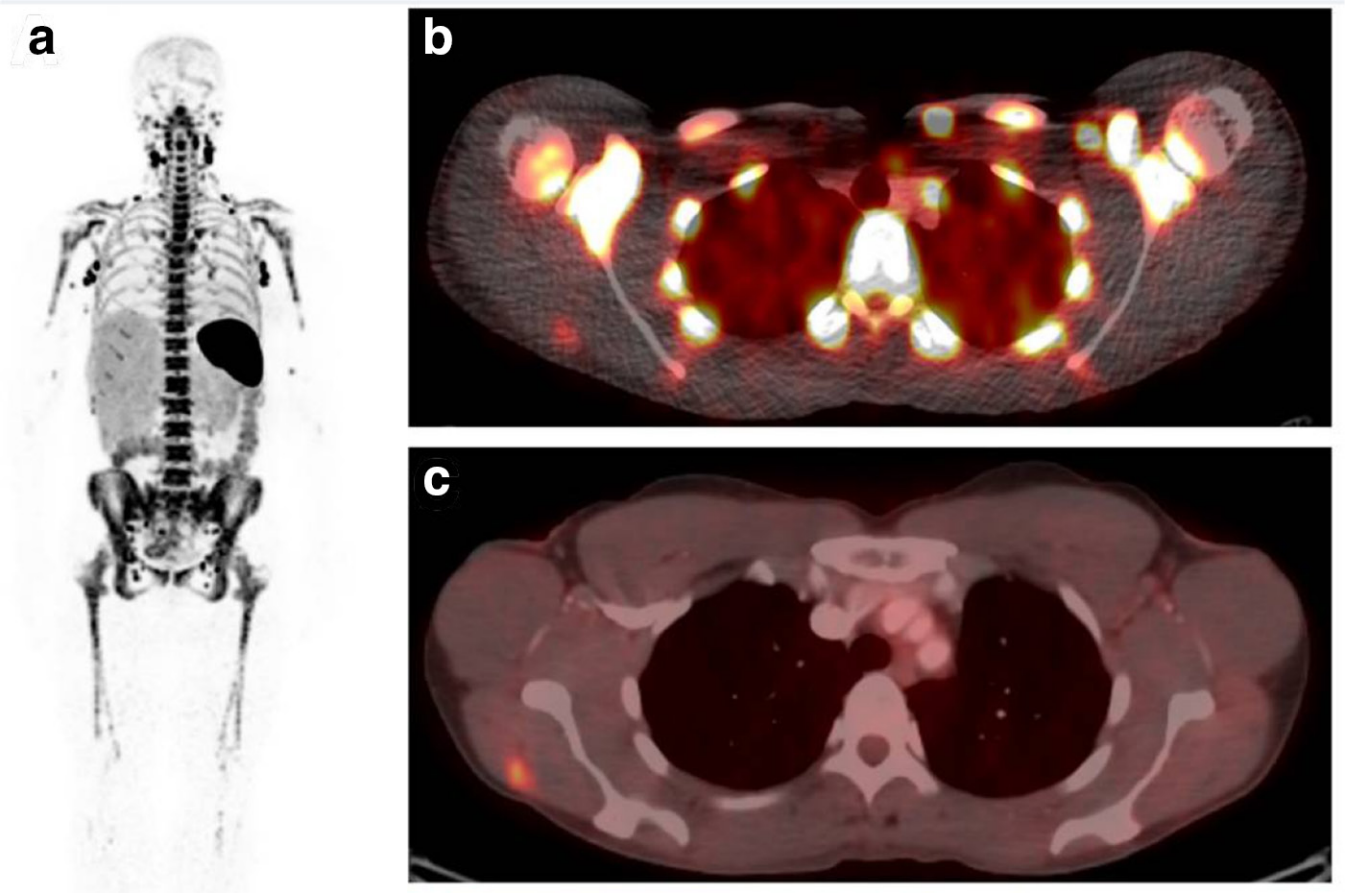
(a) Coronal projection of whole-body imaging of a melanoma patient at 24 h post-administration of ^89^Zr-IAB22M2C (CD8 minibody). Image demonstrates higher rates of uptake in the lymph nodes. (b, c) Axial fusion images of ^18^F-FDG (b) and ^89^Zr-IAB22M2C (c) at 24 h post-tracer administration. Increased uptake noted at the deltoid lesion for both tracers. This lesion could be described as “immune rich” and a site for locoregional therapy intervention in order to generate an *in situ* tumour vaccine. Images reproduced with expressed permission from JNM on behalf of Pandit-Taskar, Neeta, et al. “First-in-humans imaging with 89Zr-Df-IAB22M2C anti-CD8 minibody in patients with solid malignancies: preliminary pharmacokinetics, biodistribution, and lesion targeting.” *Journal of Nuclear Medicine* 61.4 (2020): 512–519.130
